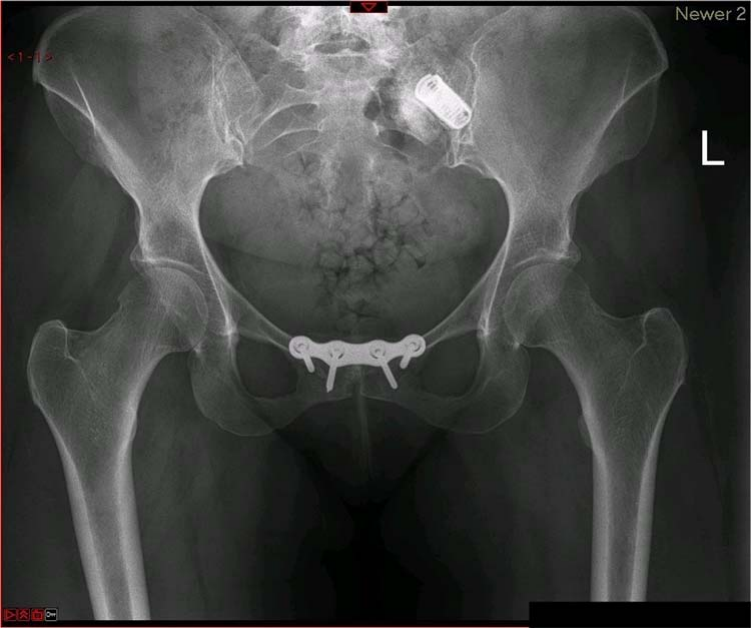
Postoperative X-ray of the pelvis following the first fusion surgery: postoperative X-ray of the pelvis, performed in November 2014 following the first fusion surgery, showing plating of the symphysis pubis and distraction arthrodesis of the left sacroiliac joint with DIANA implant.
